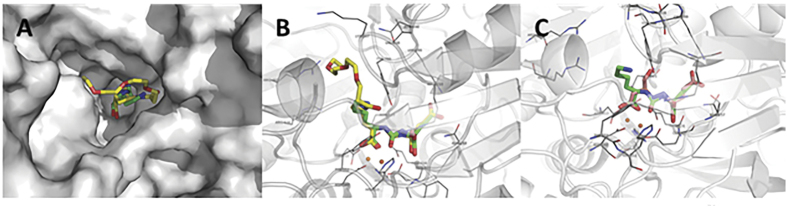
(A) PEG-DCL (yellow) and DCL (green) located in the PSMA tunnel; (B) binding modes of both PEG-DCL and DCL superimposed on the PSMA active site; (C) superimposition of DCL (green) and AG (gray) within the PSMA active site.
